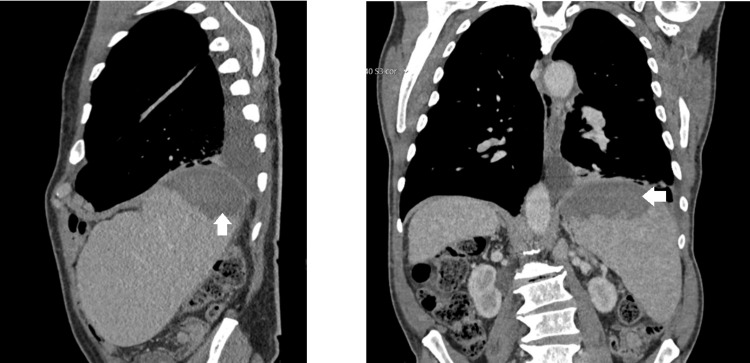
Thoracic and Upper Abdominal Computed Tomography Enlarged spleen measuring approximately 18 cm in longitudinal diameter and presenting a hypodense area at the upper pole measuring approximately 84 mm in transverse diameter, compatible with splenic infarction (white arrows)
